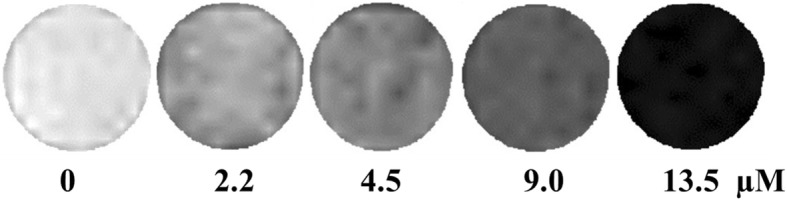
T*_2_-weighted cellular MR images of A549 cells incubated with the Fe_3_O_4_ NC/PAH/PSS/DOX hybrid nanostructures at a Fe concentration of 2.2, 4.5, 9.0, and 13.5 μM, respectively
